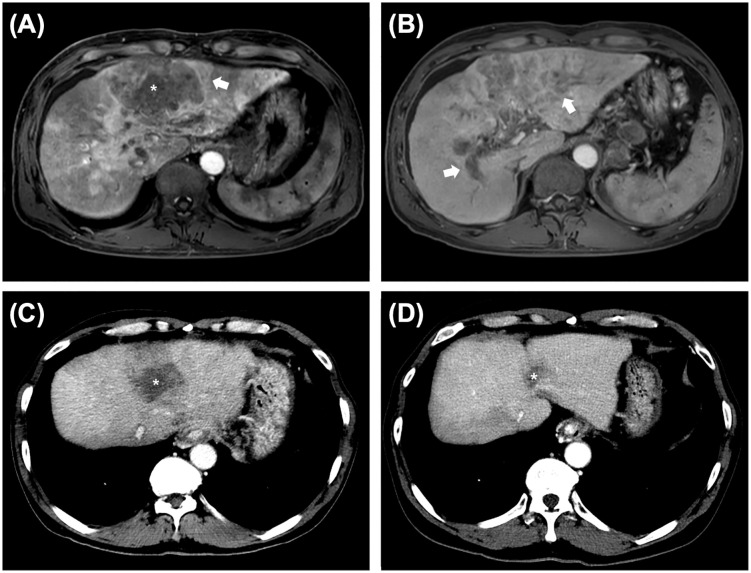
Images in a 50-year-old man with advanced hepatocellular carcinoma. (a) Arterial-phase MR image, a 9 cm sized index tumor (asterisk) with peritumoral arterial-phase hyperenhancement in liver segments III and IV. (b) Portal-phase MR reveals extensive portal vein tumor thrombosis in the left, right, and main portal veins, indicating Vp4 tumor thrombosis (white arrows). (c) Computed tomography (CT) obtained during the portal phase 3 months after atezolizumab-bevacizumab treatment without concomitant radiation therapy showed a shrunken HCC in the left hepatic lobe (asterisk) without new metastatic lesions. (d) CT obtained during the portal phase 21.5 months after the initial treatment shows an interval of more shrunken HCC in the left hepatic lobe (asterisk). The volume of extensive portal vein tumor thrombosis also decreased, and loss of its enhancement occurred with the development of cavernous transformation (not shown).
